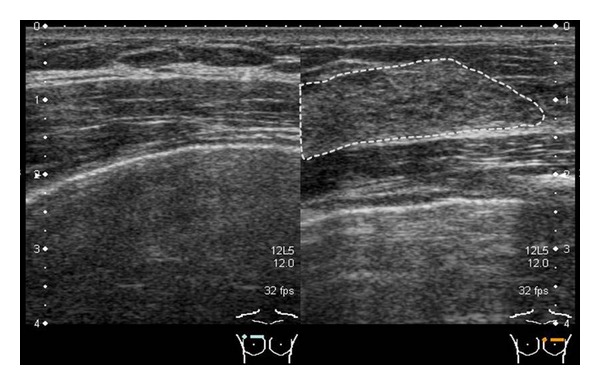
Ultrasound: thorax and pectoralis major are symmetric. Area framed by a broken line is a normal mammary gland on the unaffected side.
